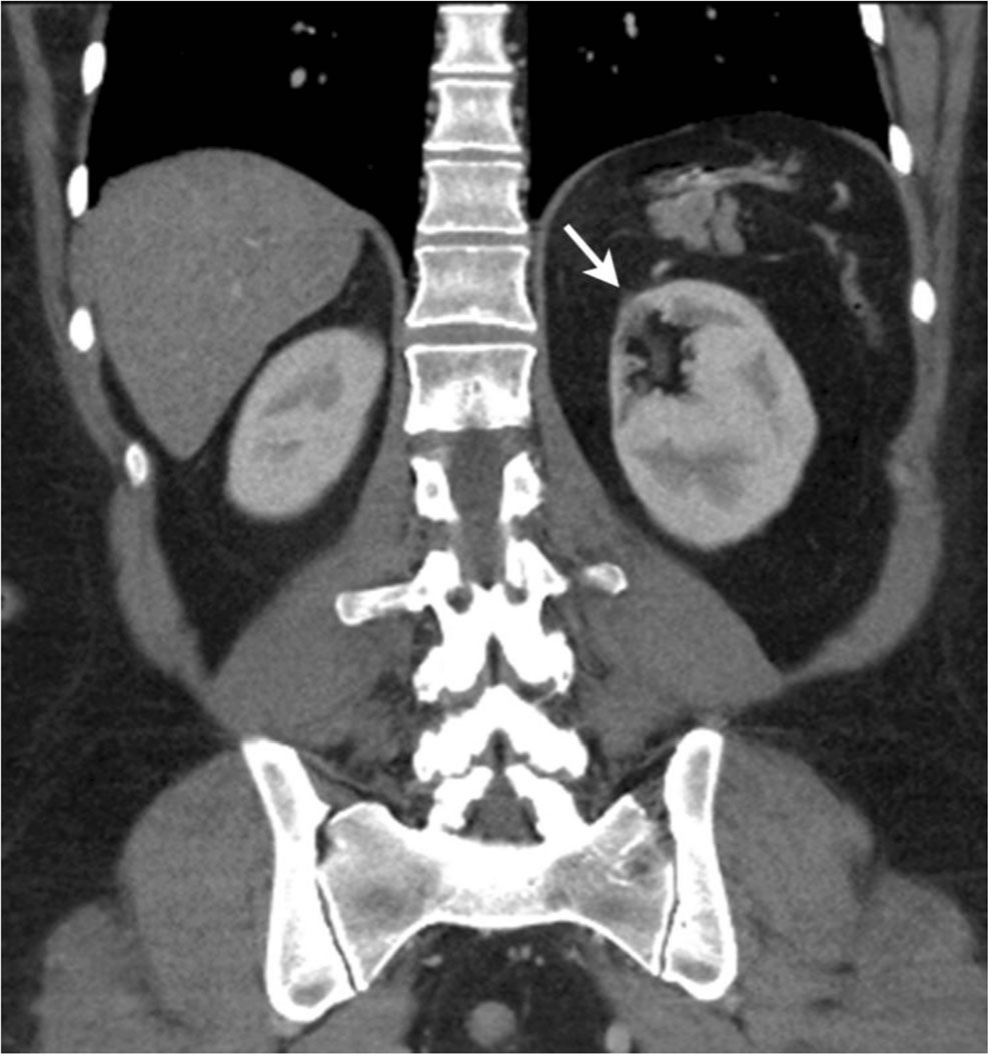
A 49-year-old female with a history of nodular sclerosing HL treated 23 years ago with mantle and paraaortic radiation to a total dose of 36 Gy. Coronal reformatted image of contrast-enhanced CT shows cortical thinning of the upper pole of the left kidney (*arrow*). Note the absence of spleen from the prior splenectomy
